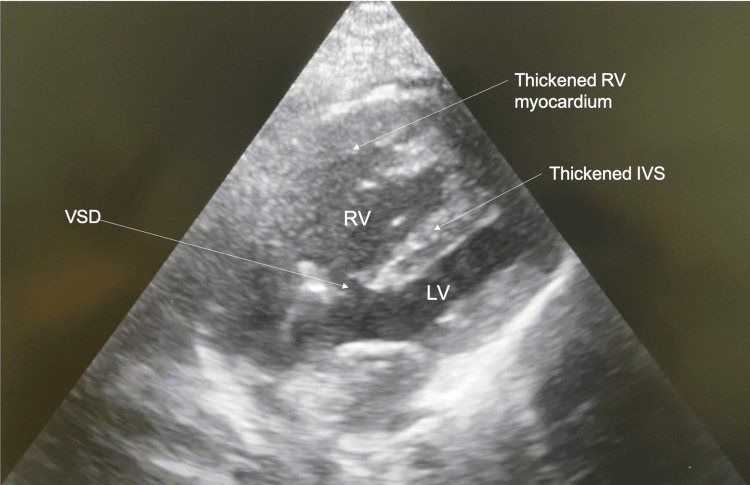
Trans-thoracic echo - apical four-chamber view LV: Left ventricle; RV: right ventricle; IVS: intraventricular septum; VSD: ventricular septal defect
